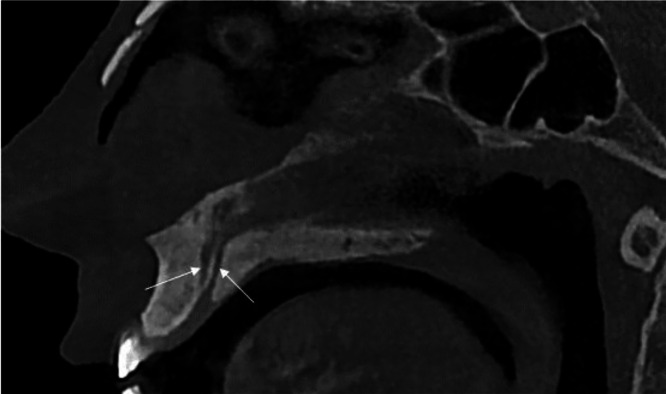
Sagittal CBCT image depicting the vertical course of the trifid nasopalatine canal, extending from the nasal floor to the incisive foramen with a funnel-shaped morphology at the oral opening. In this plane, the left and posterior canals are aligned and visible (arrows).
